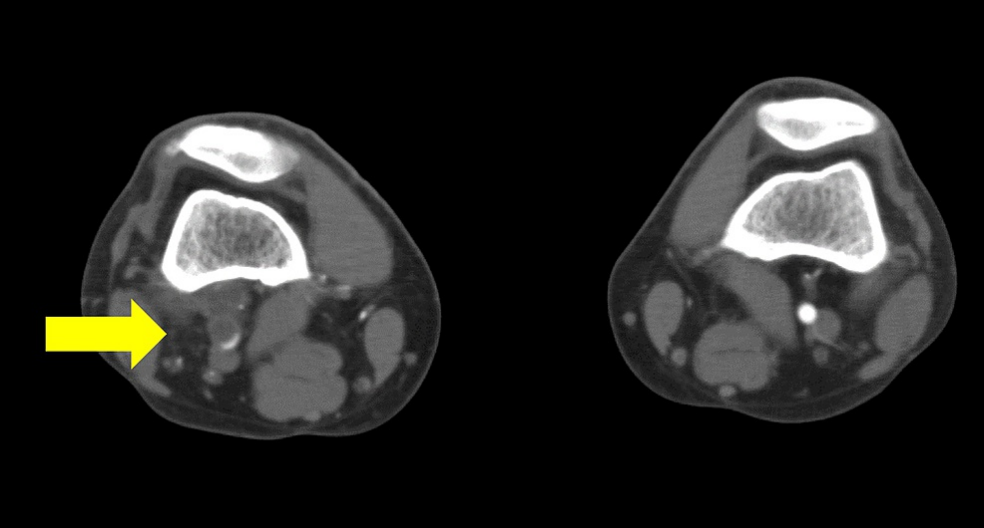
CT image CT image showing cystic adventitial disease of the popliteal artery. The arrow indicates an affected right popliteal artery, which is compressed by a non-enhancing structure surrounding the artery.
